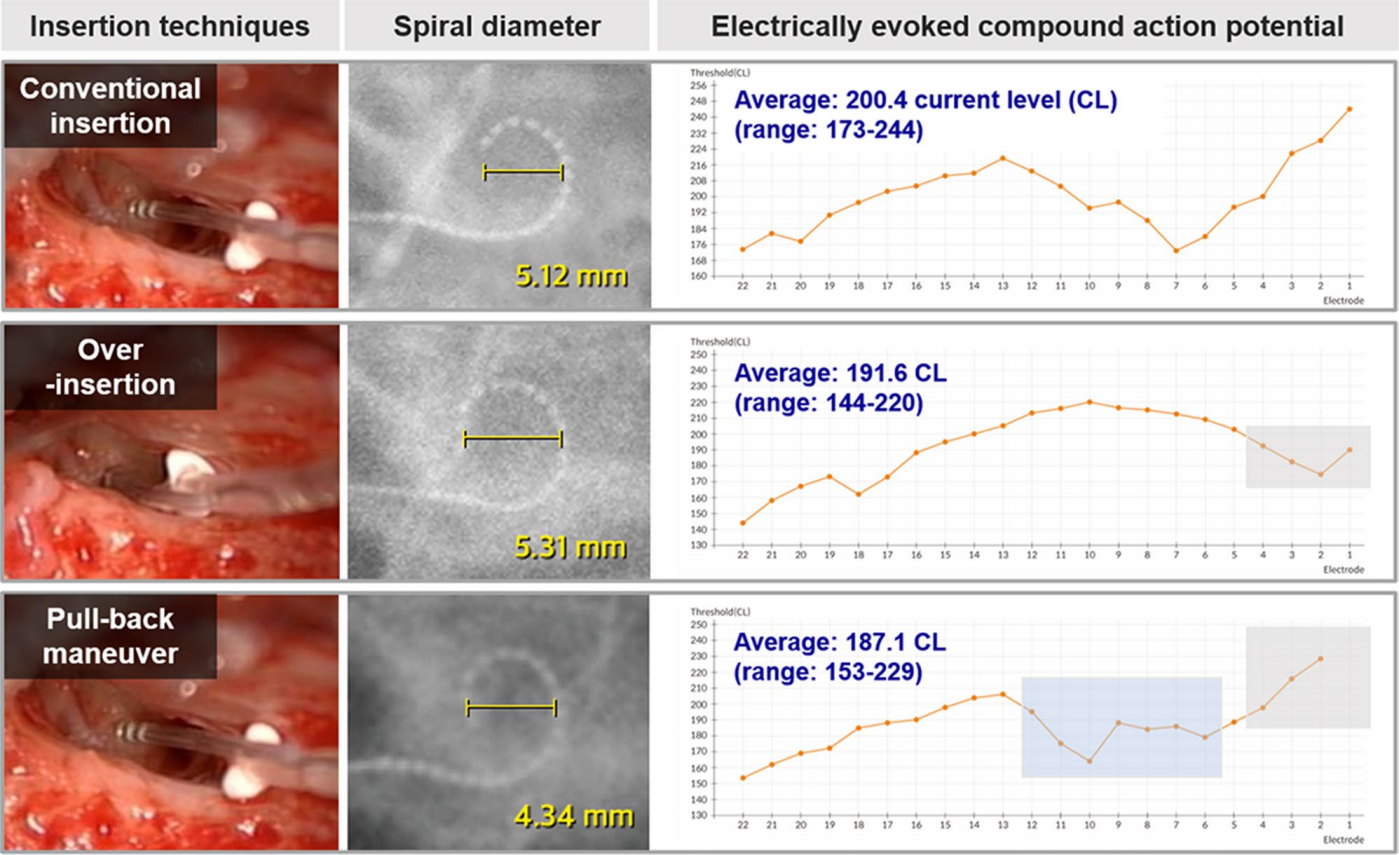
Serial changes in the spiral diameter of the slim modiolar electrode array, and electrically evoked compound action potential threshold during three insertion techniques. A representative case showing a differential spiral diameter depending on the insertion technique. This female patient is 23 months old at the time of examination and manifested bilateral deafness with congenital onset. The slim modiolar electrode arrays can be advanced or retreated with reference to three white markers of the electrode array and their relationship with the round window, facilitating a finely tuned insertion technique. Specifically, the pull-back maneuver-assisted insertion led to a significantly shorter spiral diameter based on intraoperative simple X-ray (i.e., cochlear view), compared with conventional and over-insertion. However, the angular insertion depth remained unchanged even after the pull-back maneuver-assisted insertion, compared with over-insertion. During the procedure, over-insertion led to reduced ECAP thresholds of the last four basal electrodes (Electrode 1–Electrode 4, gray rectangular box), compared with conventional insertion. Subsequently, pulling-back the electrode significantly reduced thresholds for the upper basal and middle electrodes (Electrode 6–Electrode 12, blue rectangular box), while again elevating ECAP thresholds of the last four basal electrodes (Electrode 1–Electrode 4, gray rectangular box) similar to the those of conventional insertion.
